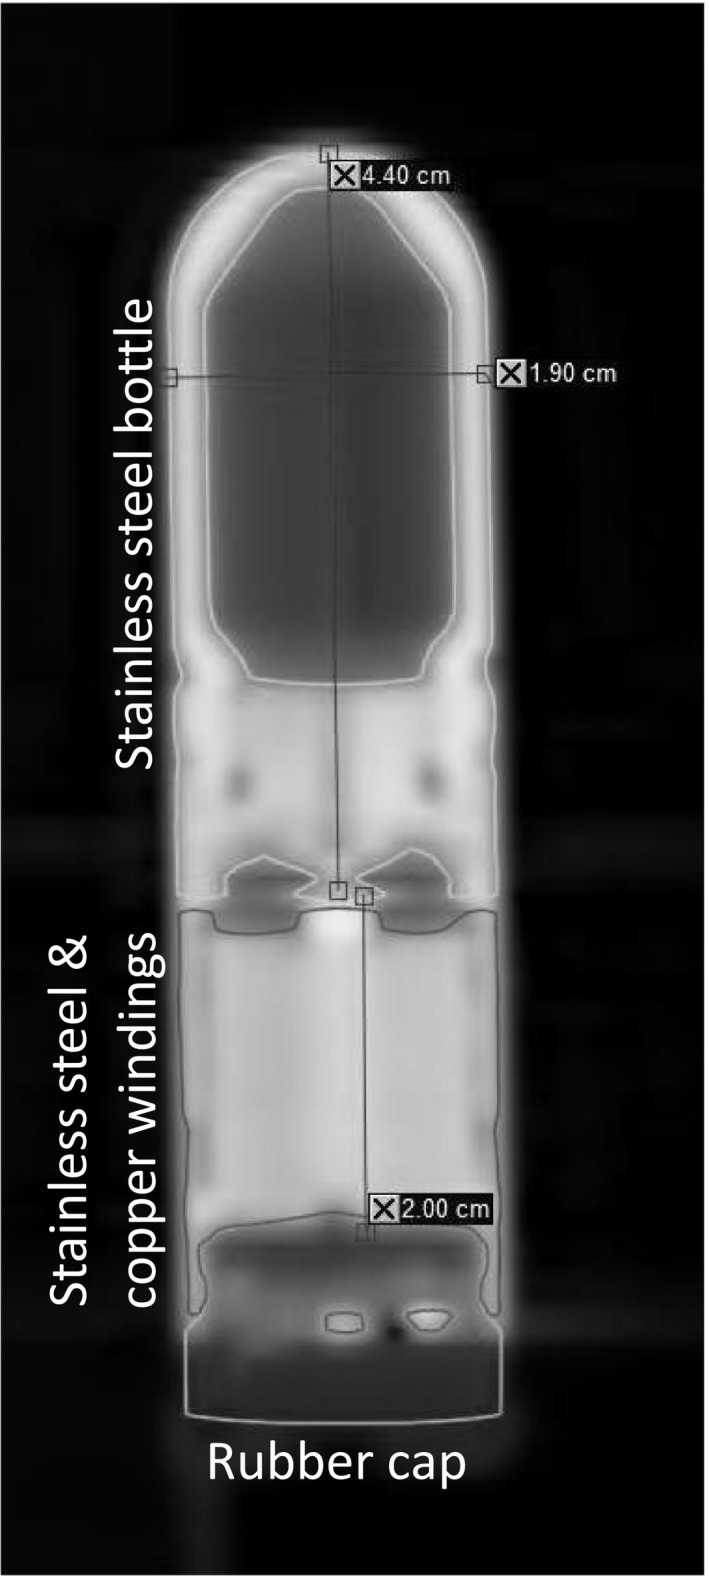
Coronal CT slice of the 400–600 cc AeroForm CO_2_ reservoir using optimal geometry and acquisition technique. Individual components are defined and verified with manufacturer specifications.
